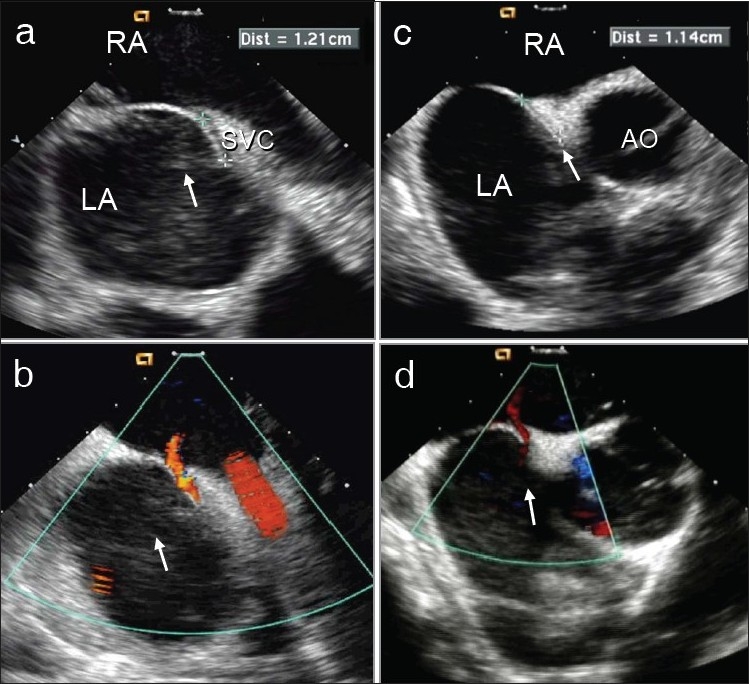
Intracardiac echocardiography documenting two standard views. (a,b) is the bi-caval view without and with color, respectively. This is a tunnel PFO with significant overlap of the septum primum on the septum secundum (the SVC rim). The measurements in the picture is of the length of the tunnel. (c,d) Aortic short-axis view without and with color, respectively. Aortic rim is seen with septum primum overlapping the septum secundum (the aortic rim).
